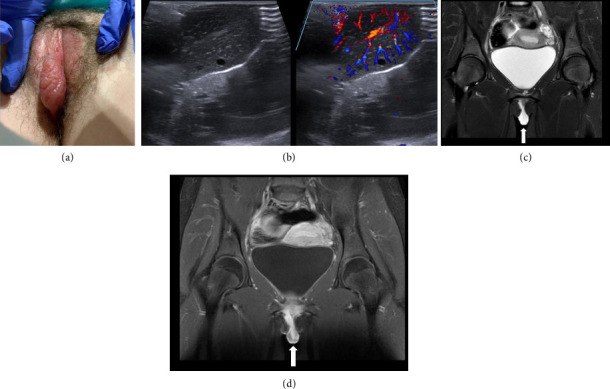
Patient 2 examination and imaging (ultrasound and MRI) findings. (a) Imaging findings on ED presentation. (b) Sagittal high-resolution ultrasound demonstrating a solid right labium mass with hyperemia on color Doppler imaging. (c) Coronal T2-weighted MRI demonstrating a hyperintense right labium mass (arrow). (d) Postcontrast coronal T1-weighted image with fat saturation shows intense heterogeneous enhancement of the mass (arrow).
